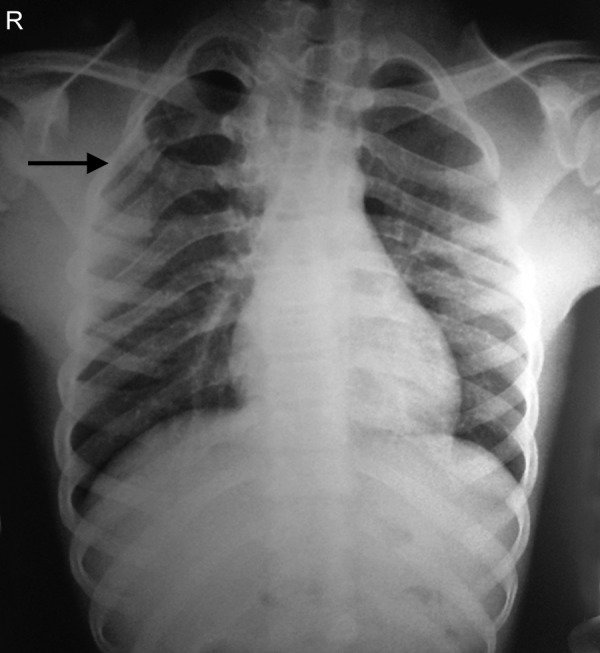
PA view chest X-ray showing vertebral rib deformity in the cervicodorsal region
